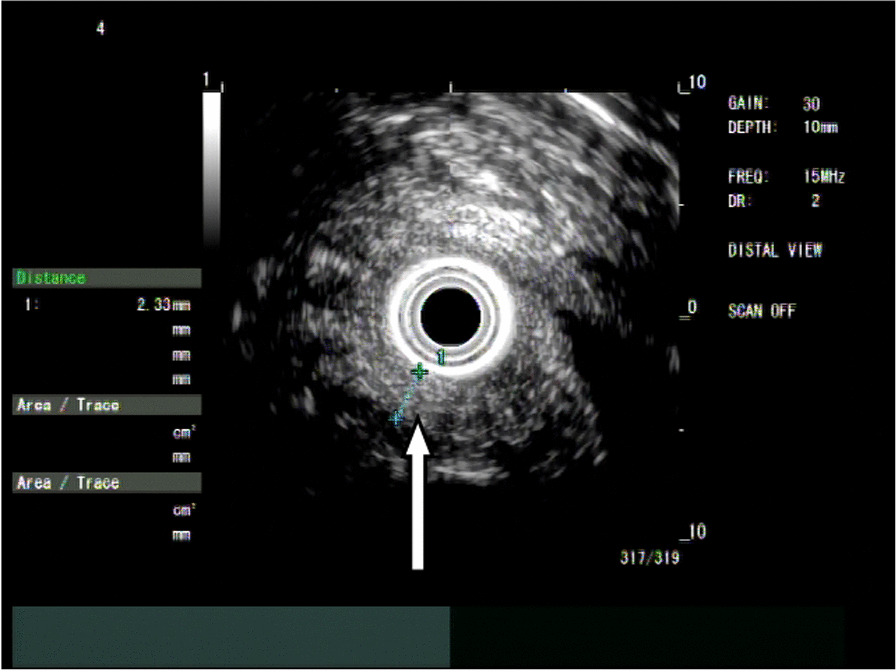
Microprobe of ultrasonic endoscope scaned the scar of esophageal stricture
